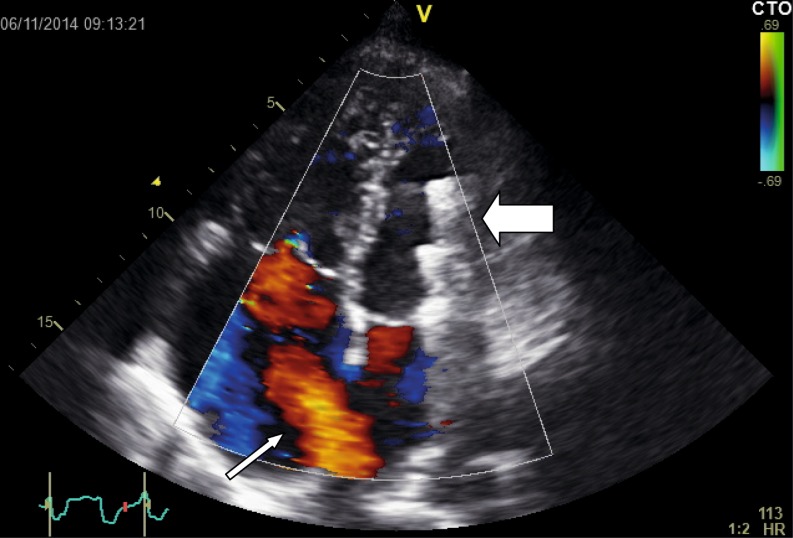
Transthoracic echocardiogram from a patient with an ASDII, showing clear left-to-right shunting through an open defect (*small white arrow*) and right-atrial enlargement. The previously implanted closure device has embolised to the left ventricle (*large white arrow*)
